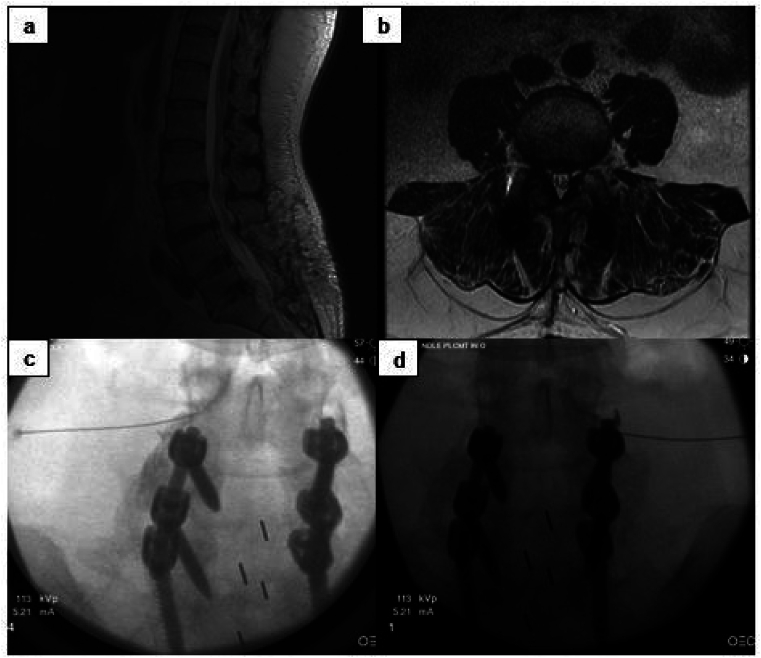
a) Sagittal T2-weighted MRI in patient with previous laminectomy and fusion b) Axial T2-weighted MRI at L3-4 in same patient c) Transforaminal epidural steroid injection at left L3-4 d) Transforaminal epidural steroid injection at right L3-4 in same patient.
